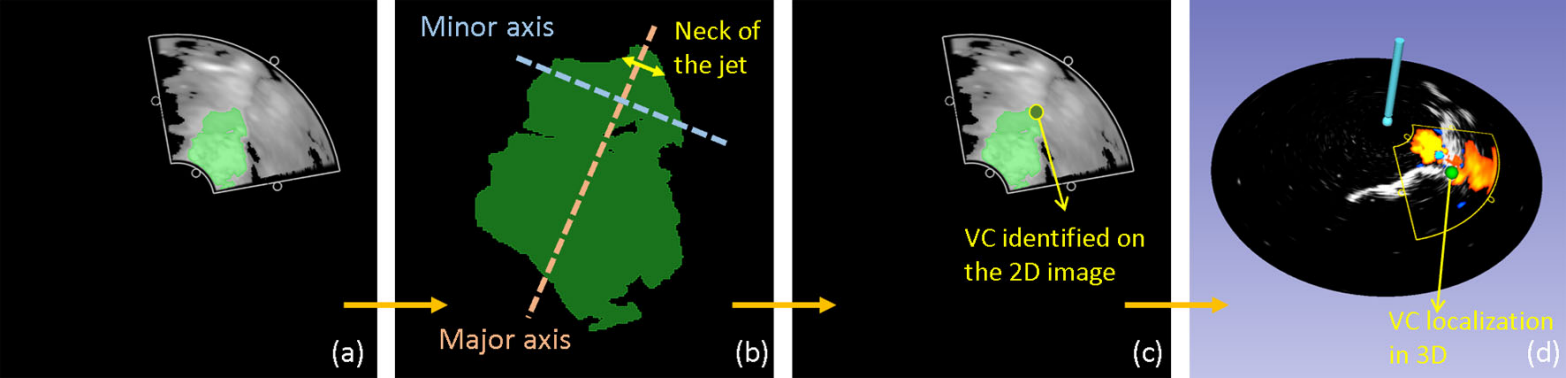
a Resultant Doppler image overlaid with the segmentation of the regurgitant jet. b Principal component analysis of the segmented region to derive the location of the vena contracta (VC). VC localization seen on c a 2D image and in d 3D tracking space
